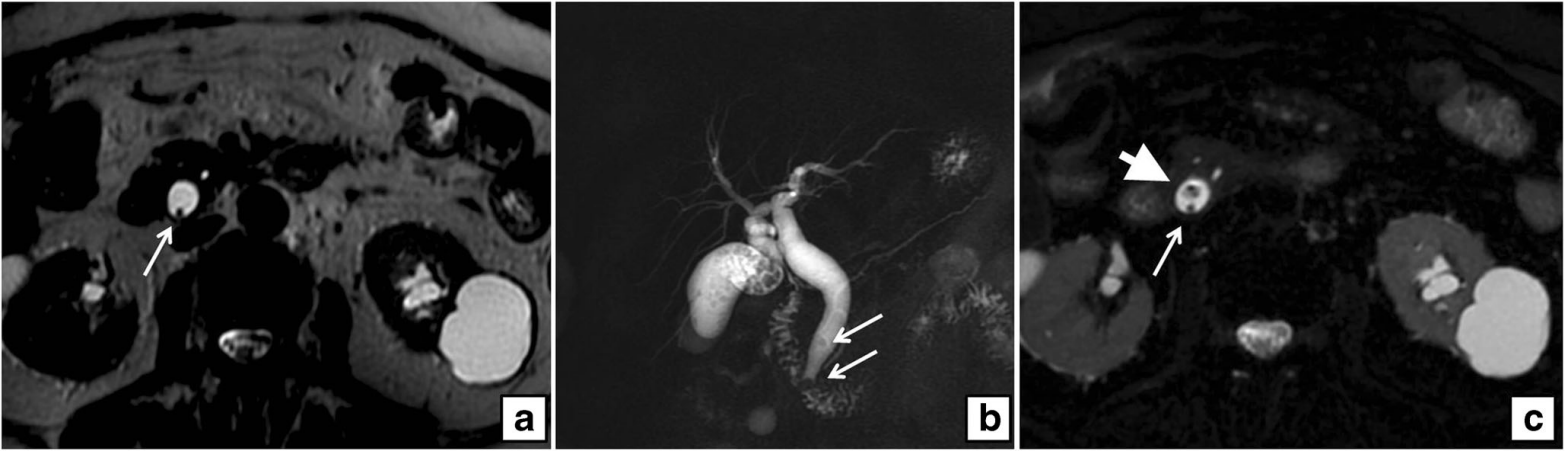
a Axial T2-weighted MRI shows a millimetric filling defect in a dilated CBD (*arrow*). b Coronal MIP reformat of the same patient shows multiple filling defects in the gallbladder and two stones are seen in the distal CBD (*arrows*) with mild upstream dilatation. c Axial fat-saturated T2-weighted MRI of the same patient shows two filling defects in a dilated CBD (*arrow* and *arrowhead*) at the same level of a
